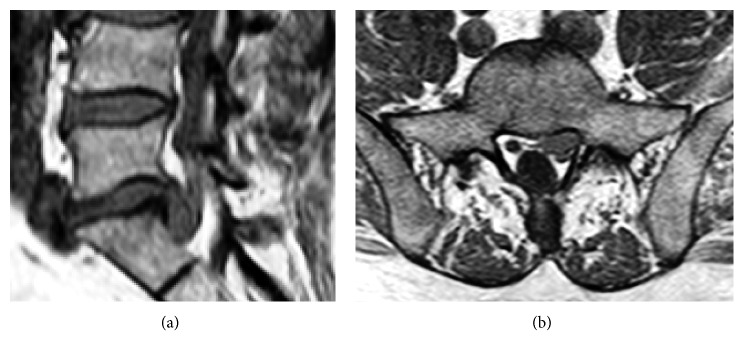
Lumbar MRI of case 2 with large left side caudally dislocated herniation.
